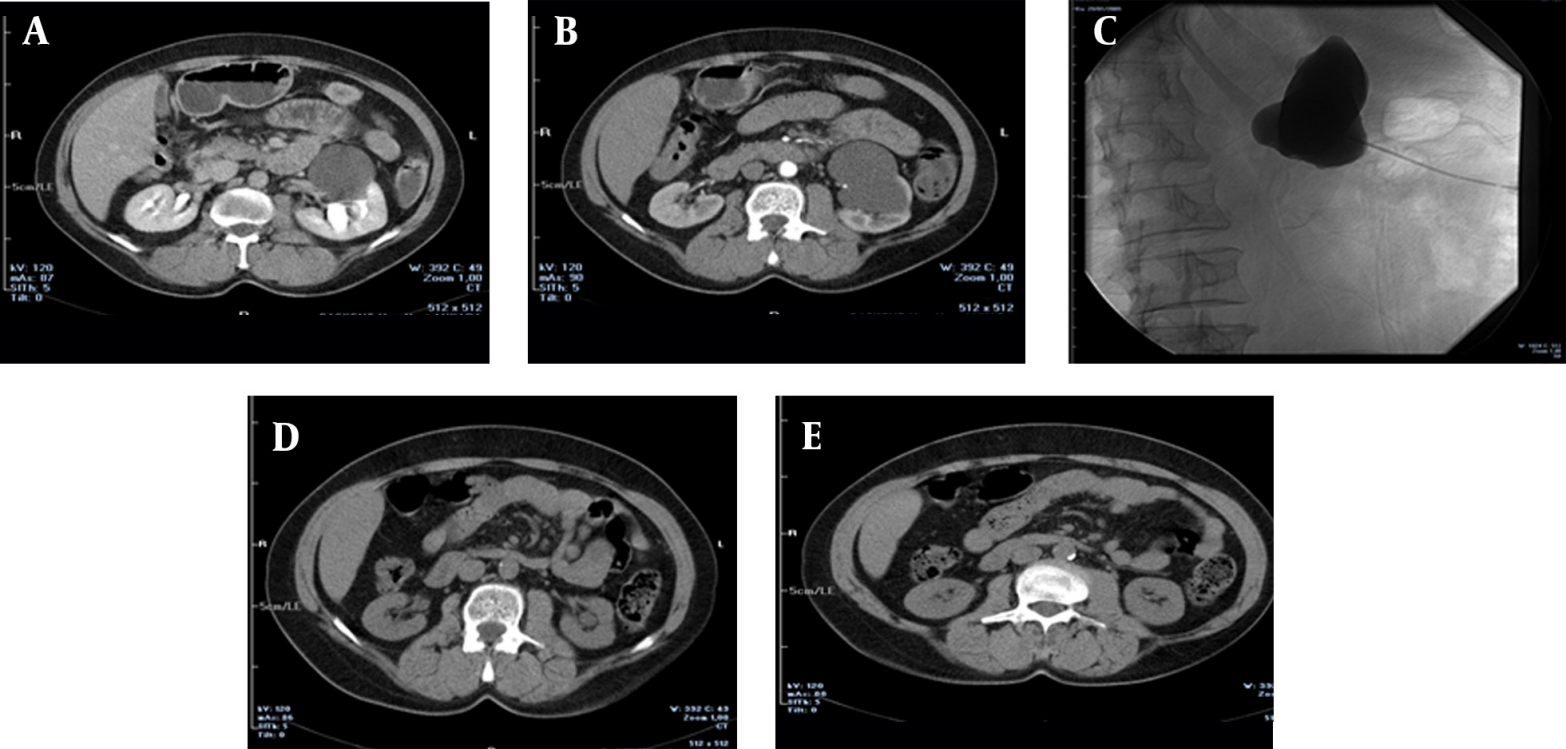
A 72-year-old man suffering from left flank pain for 6 months, A and B) IV contrast enhanced CT images show the cyst located at the lower portion of the left kidney which is classified at Bosniak type-1; C) Cystogram image shows that the cyst does not connect with the pelvicalyceal system; D and E) CT images obtained 3 months after the treatment show that the cyst is completely regressed and the patient had no pain.
